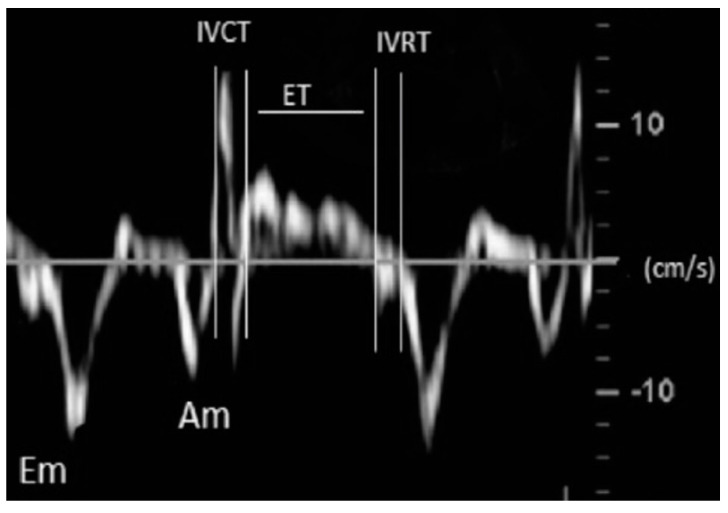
This figure shows a Doppler tissue imaging obtained at the mitral annulus for the calculation of the TEI index as a parameter for the estimation of the LV function in this study. IVCT (isovolumetric contraction time); IVRT (isovolumetric relaxation time); ET (ejection time).
